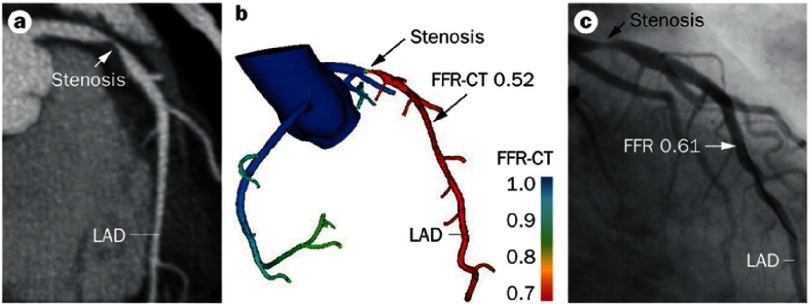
(a) Computed tomography (CT) images of a stenosis in the left anterior descending artery (LAD), (b) Fractional flow reserve (FFR) measured by CT and, (c) the corresponding images and FFR in invasive catheter.
